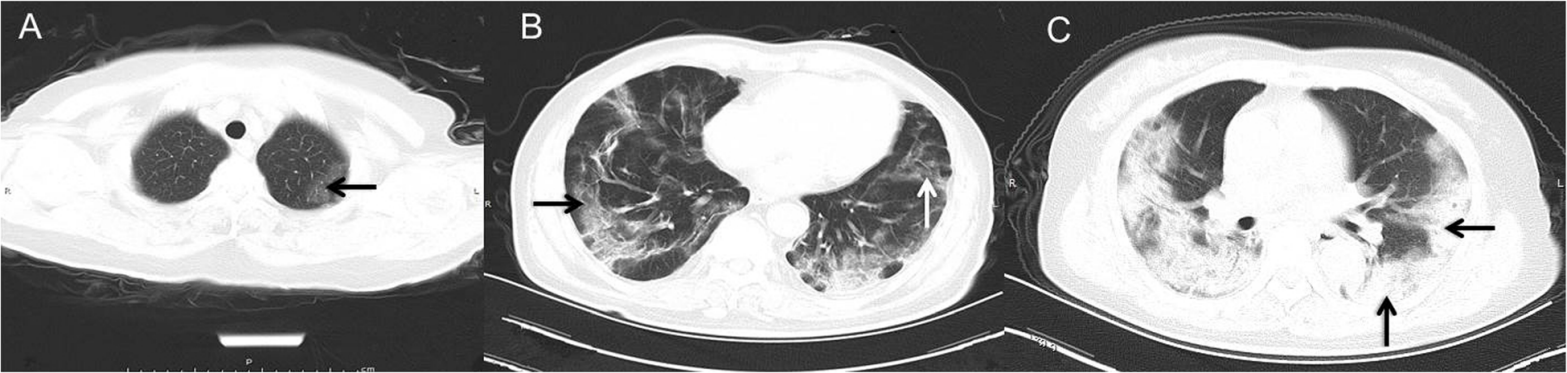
Representative lung CT images of patients who had frosted glass density (a), ground glass opacity (b), and consolidation (c)
